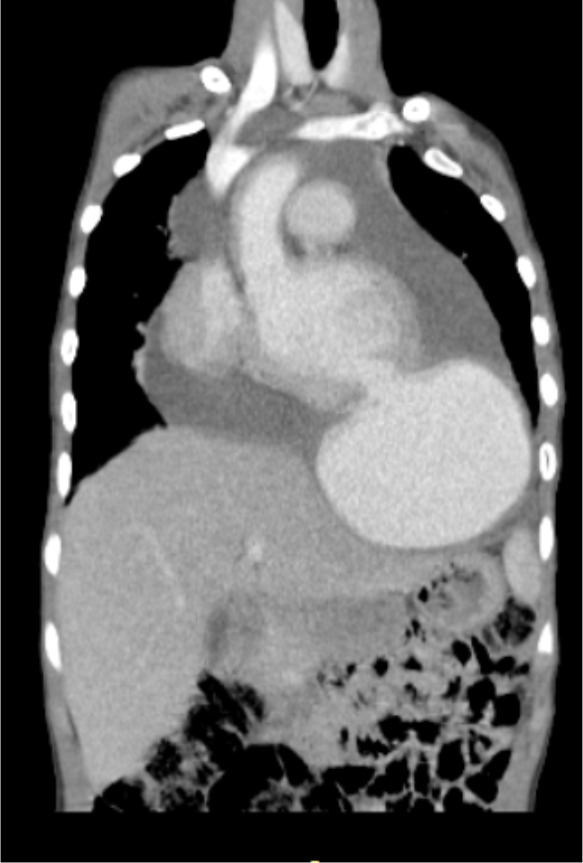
CT-scan of the heart with a communication of the left ventricle with aneurysm
